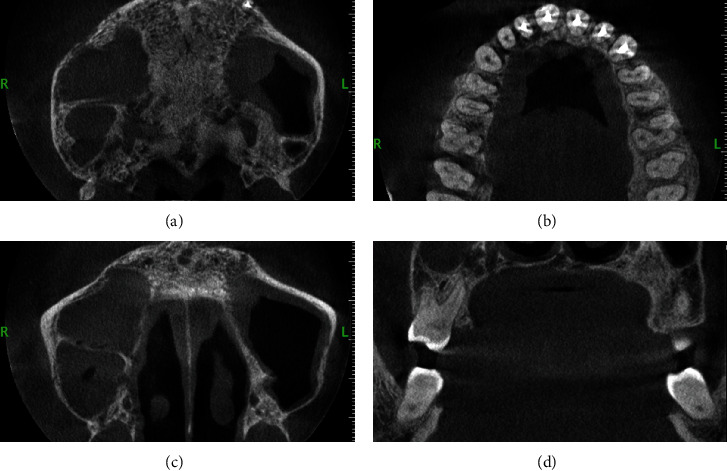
CBCT of case 1: (a–c) axial views; (d) cross-sectional view. (a) Osteolytic change in the posterior area. (b) Radiopaque obturating material in maxillary anterior teeth with resorption of alveolar process. (c) Haziness in the maxillary sinus on the right side. (d) Osteolytic changes on the palatal alveolar region on the maxillary 1^st^ molar.
